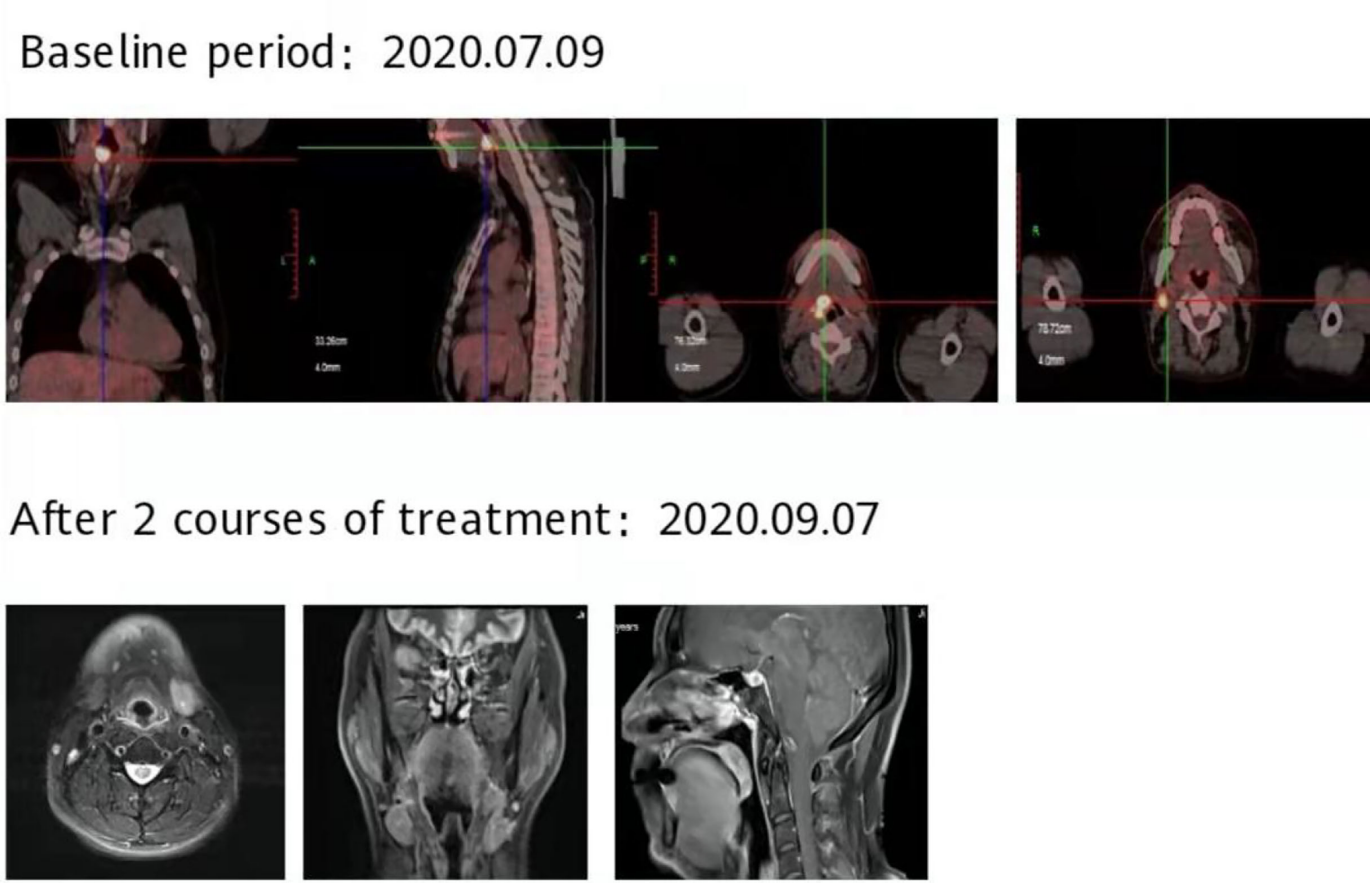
After 2 courses of treatment, no lesions were found in the MRI of the throat, and the curative effect was evaluated as complete response (CR).
